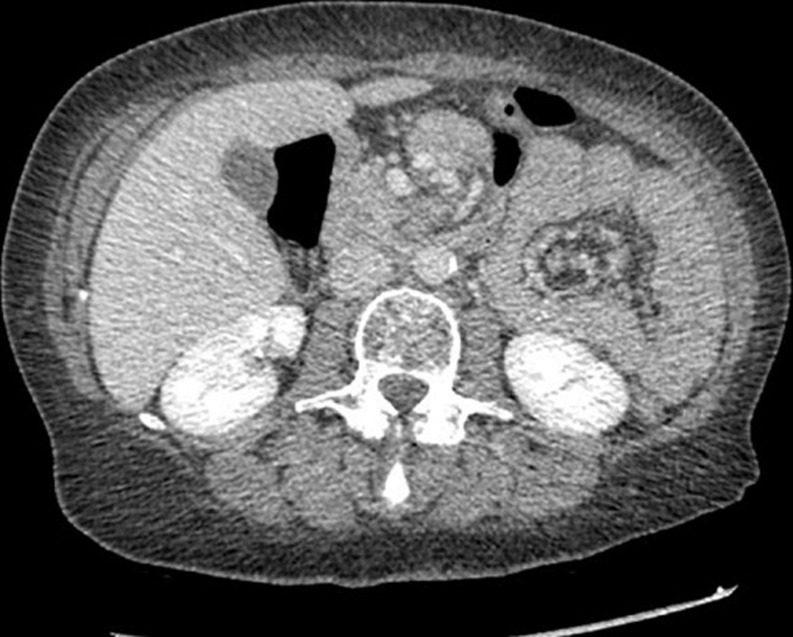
CT scan demonstrated a “twist” in the mesentery with “whirpool sign” of the jejunum
